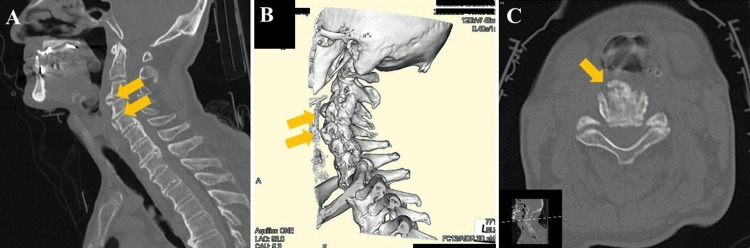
CT imaging (A) Sagittal CT image. (B) 3D CT image. (C) Axial CT image. Evidence of ossification of the anterior longitudinal ligament at the C3/4 and C4/5 is observed (yellow arrow). CT: computed tomography.
